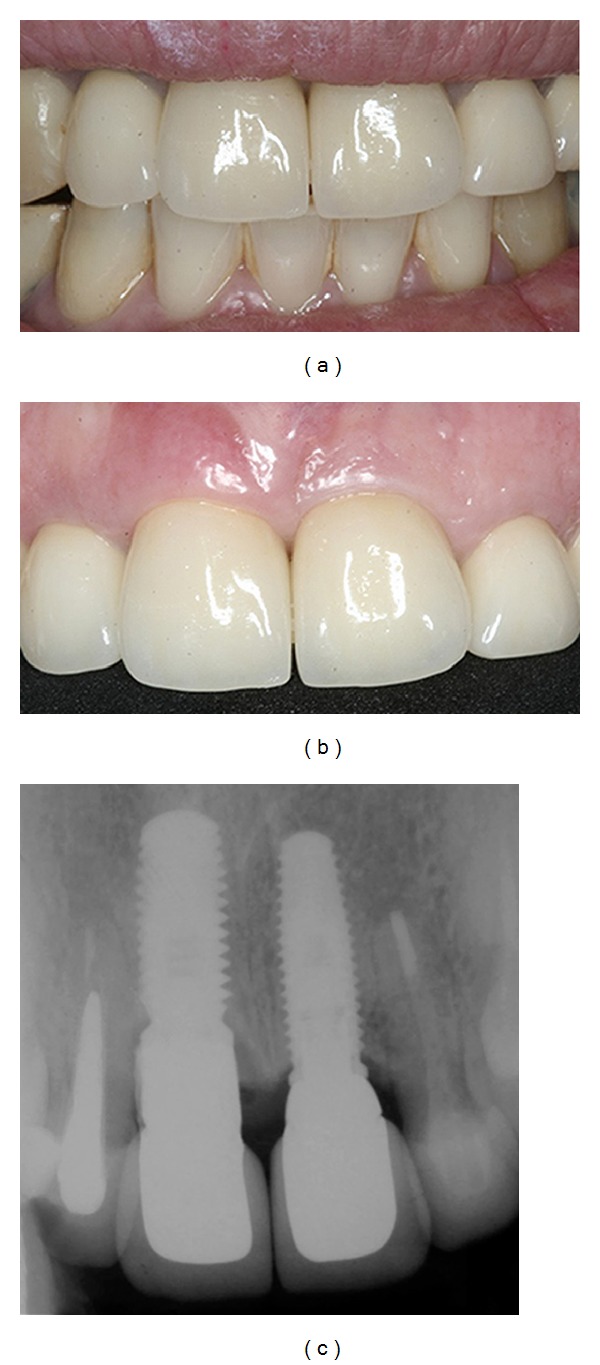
(a, b) Three years follow-up; the result showed esthetic excellence of the definitive prosthesis; (c) periapical radiograph. Three-year follow-up showing the accurate fit between the abutments and implants and between the crowns and abutments.
